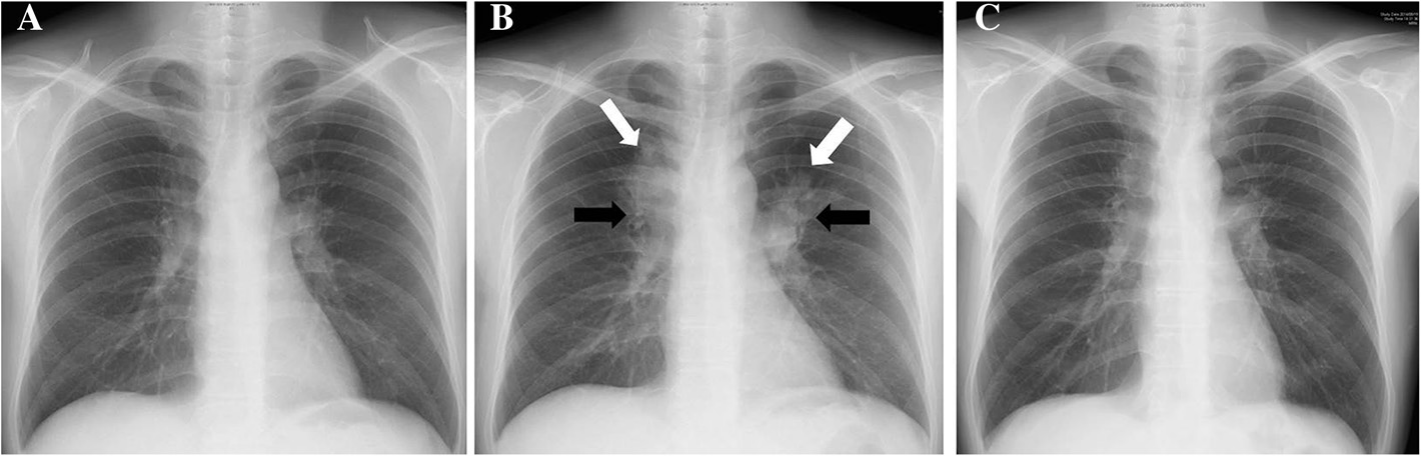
Serial changes on chest radiograph. a Chest radiograph taken at the time of starting combined antiretroviral therapy (cART), showing almost normal findings. b Chest radiograph taken five months after starting cART, showing infiltrates in the bilateral upper lung fields (white arrow) and bilateral hilar lymphadenopathy (black arrow). c Chest radiograph taken one year after starting anti-mycobacterial chemotherapy, showing slight hilar lymphadenopathy
